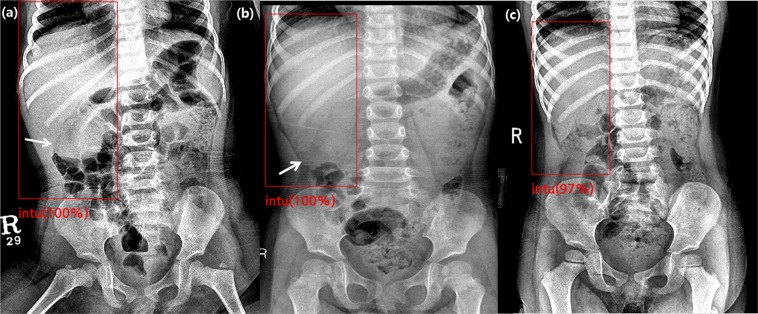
True-positive cases found by the algorithm in the validation set. (a) A supine abdominal radiograph of a 26-month-old female with abdominal pain. Her initial radiograph shows a round opacity in the right upper abdomen (arrow) and the ileocolic intussusception was confirmed on US. To train the algorithm, an ROI using the upper boundary of the right diaphragm, lower boundary of the iliac crest, medial boundary of the spine, and lateral boundary of the lateral rib cage was drawn. This image was actually included in the validation set. The algorithm and two out of four radiologists correctly diagnosed intussusception, while the other two radiologists suggested it negative for intussusception. (b) An abdominal radiograph of a 28-month-old female with intussusception. One radiologist labeled it positive for intussusception based on the round opacity in the right upper abdomen (arrow) and the algorithm reported a true-positive result. (c) An abdominal radiograph of a 30-month-old girl with intussusception. The algorithm reported a true-positive result, while all four radiologists reported false-negative results.
